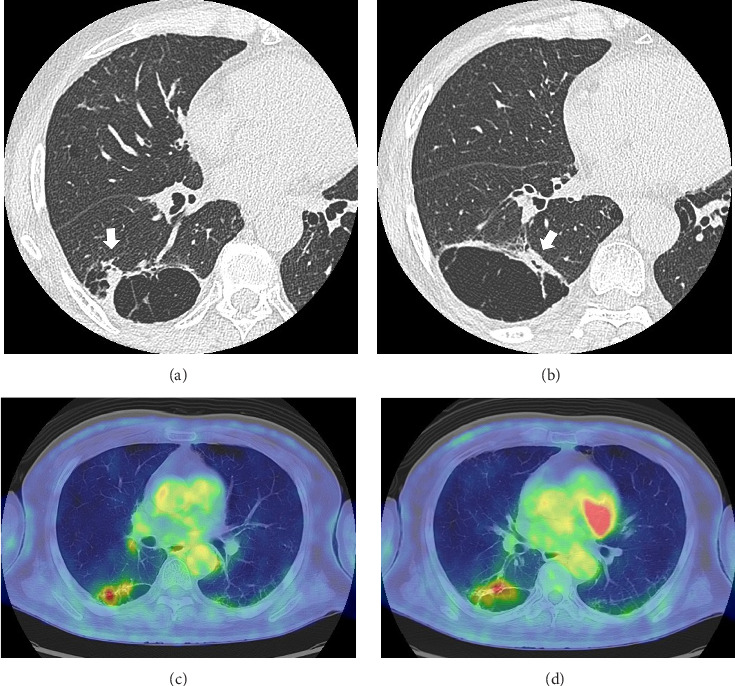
High-resolution computed tomography (HRCT) (a and b), and 18F-fluorodeoxyglucose positron emission tomography/CT (c and d) images 14 months after initial chemotherapy showing disseminated thickened-walled cystic airspaces and two enlarged nodules (arrows) measuring 1.2 cm in the right lower lobe with a high 18F-FDG uptake.
